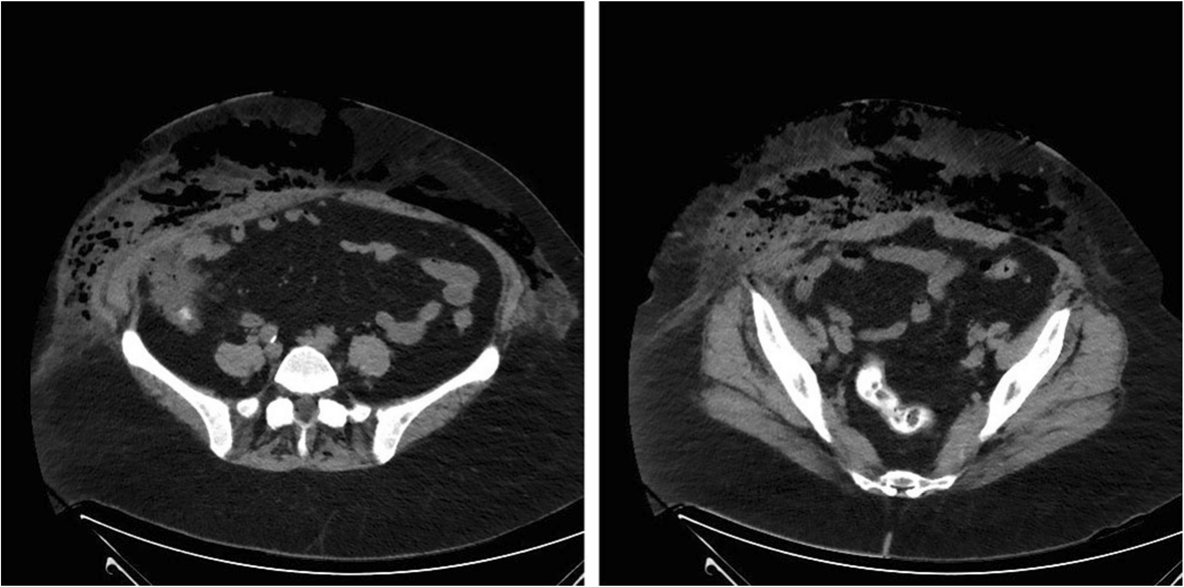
Computed tomography performed on the patient’s admission shows extensive emphysema in the subcutaneous tissue without any signs of an intraabdominal infection
